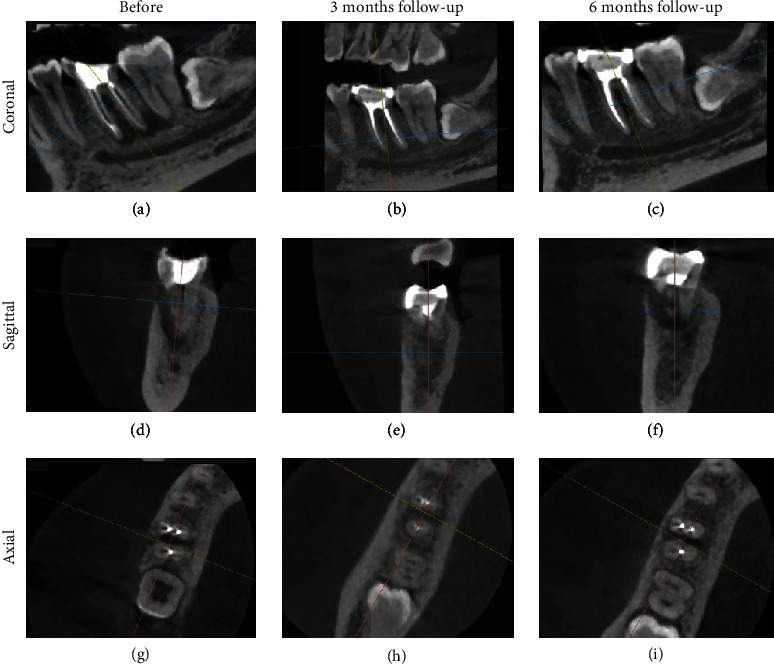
CBCT (a, d, g) before periodontal treatment, (b, e, h) 3 months, and (c, f, i) 6 months of follow-up arranged sequentially, consisting of coronal, sagittal, and axial views.
